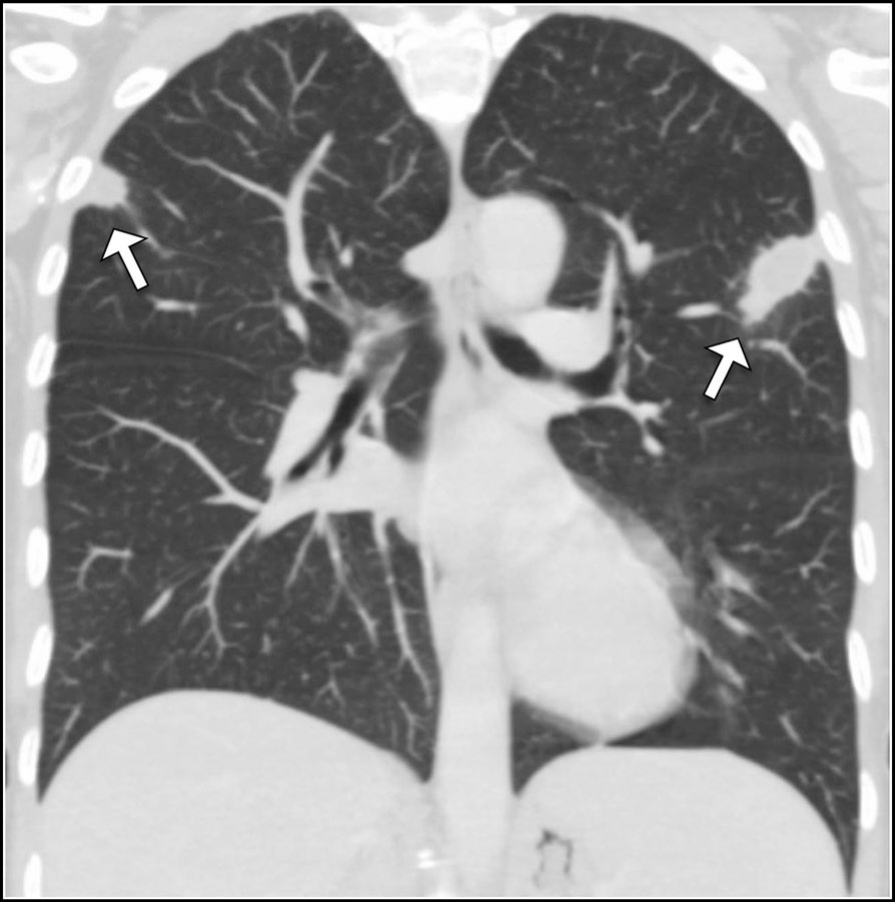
Coronal CT image of the chest shows peripherally located wedge-shaped consolidations (arrows) in the upper lobe of both lungs. These findings support the diagnosis of septic emboli in the presented case of Lemierre syndrome
